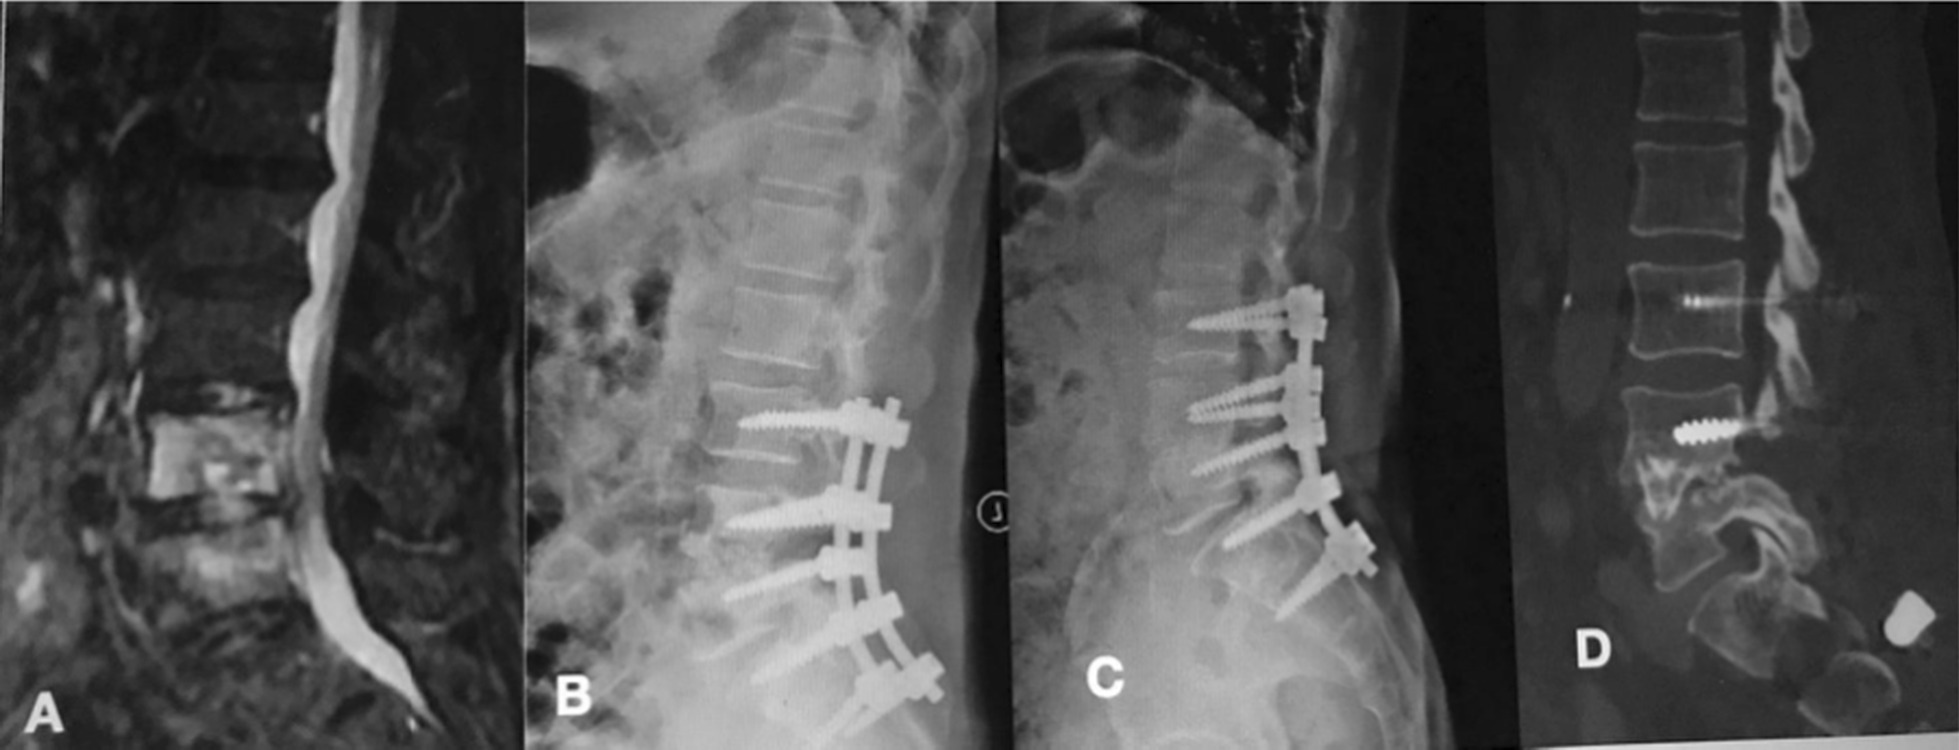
A patient aged 30–40 years old with lumbar pyogenic discitis (L4–5) underwent single-segment posterior lumbar interbody fusion combined with instrumentation with an LSP. A Pretreatment MRI showed the destruction of the L4–5 disc and concomitant compression of the spinal nerves. B Twelve-month postoperative X-rays showed maintained correction. C, D At the 32-month FU, plain X-ray and CT showed the good correction and reliable fusion
